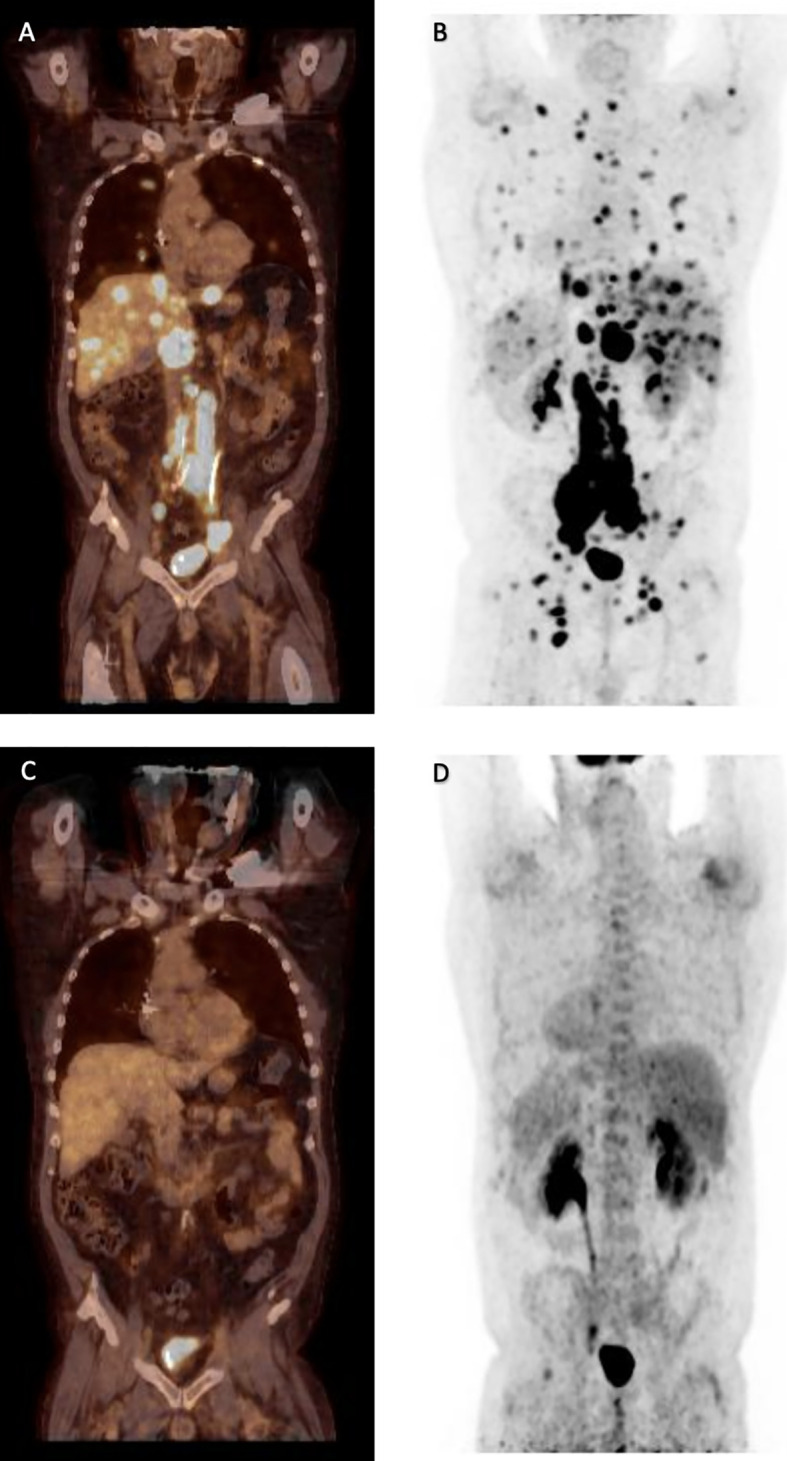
Positron emission tomography with (A, C) and without computed tomography co-registration (B, D) in ventral (A, C) and dorsal view (B, D). Disseminated manifestations of diffuse large B-cell lymphoma refractory to chemoimmuntherapiy prior to CAR-T cell therapy (A, B) and in remission three months after anti-CD19 CAR-T treatment with axi-cel.
